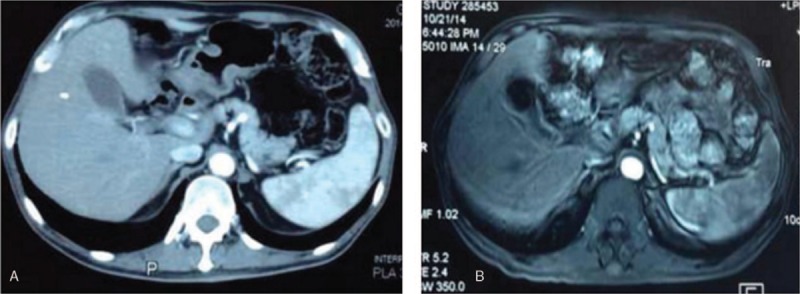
After combination treatment, abdominal computed tomography (A) and abdominal magnetic resonance imaging (B) revealed a small cyst in the right anterior lobe of the liver but no metastatic liver tumor.
